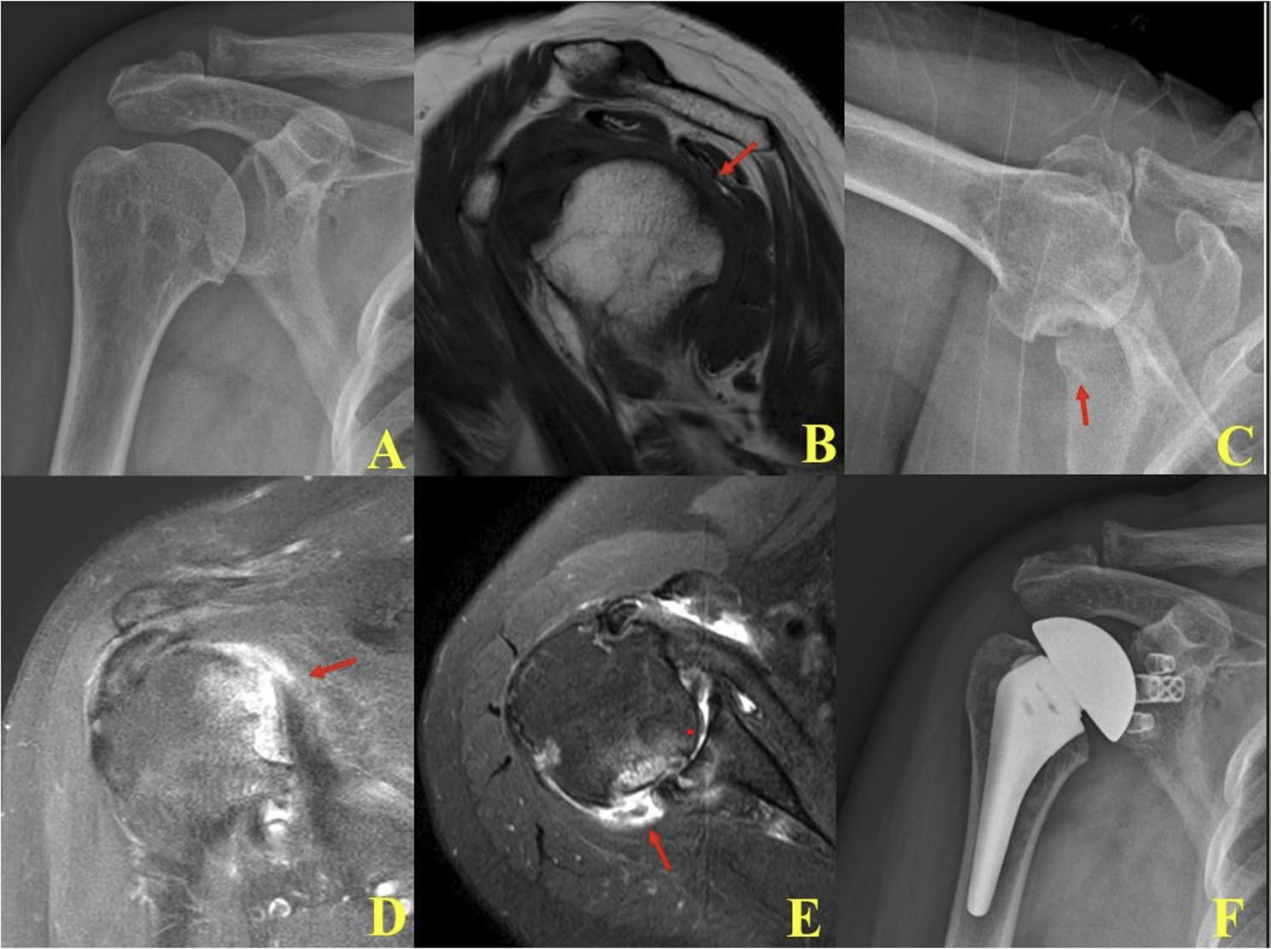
In the early prodromal phase of RPDA: A Initial plain radiograph showed no specific abnormalities of the humeral head. B Initial MRI revealed focal bone marrow edema, rather than diffuse involvement of the entire humeral head. C At six-month follow-up, plain radiograph demonstrated a newly developed focal subchondral fracture of the humeral head. D, E Follow-up MRI at six months confirmed the subchondral fracture without evidence of glenoid defects or rotator cuff tears. F Total shoulder arthroplasty was subsequently performed
